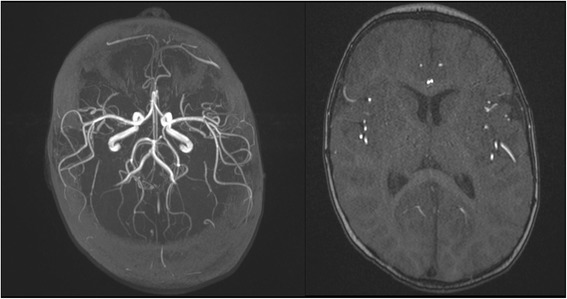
3D Time of flight angiography (TOF) MRI sequence, transverse plane
